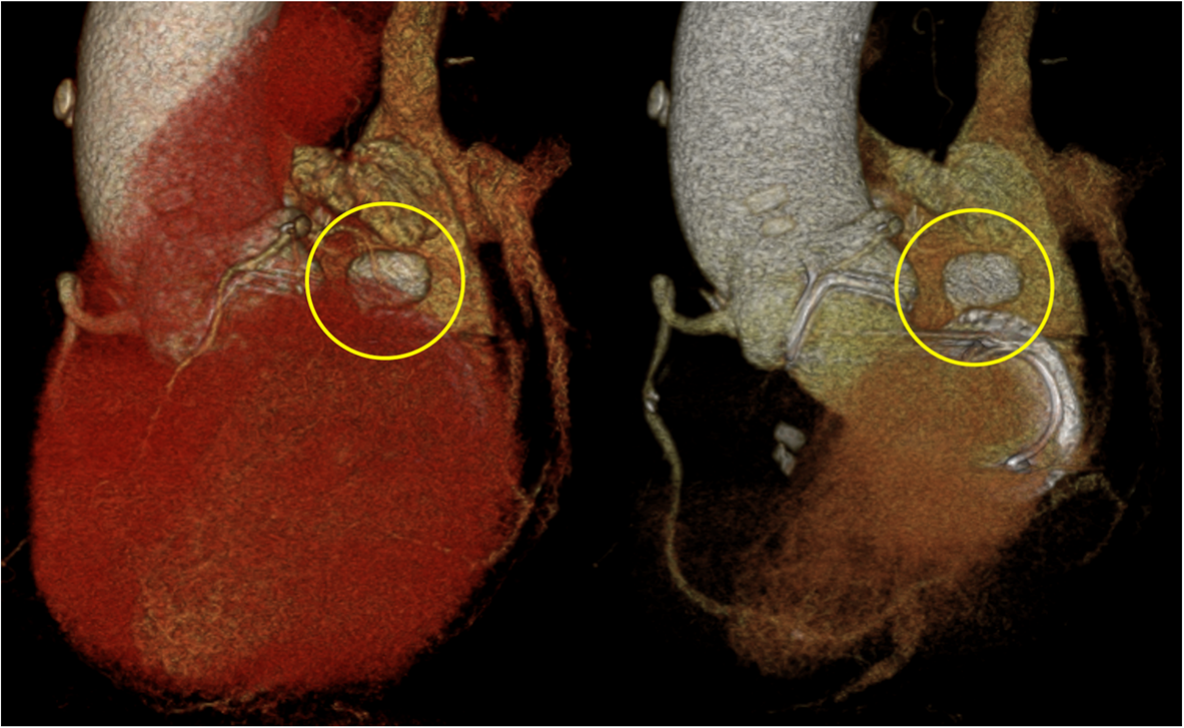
Left ventricular pseudoaneurysm following intraoperative atrioventricular groove hematoma. Volume rendering cardiac computed tomography on postoperative day 18 shows a pseudoaneurysm (20 × 12 mm) arising from the posterior wall of the LV just below the implanted mitral valve
